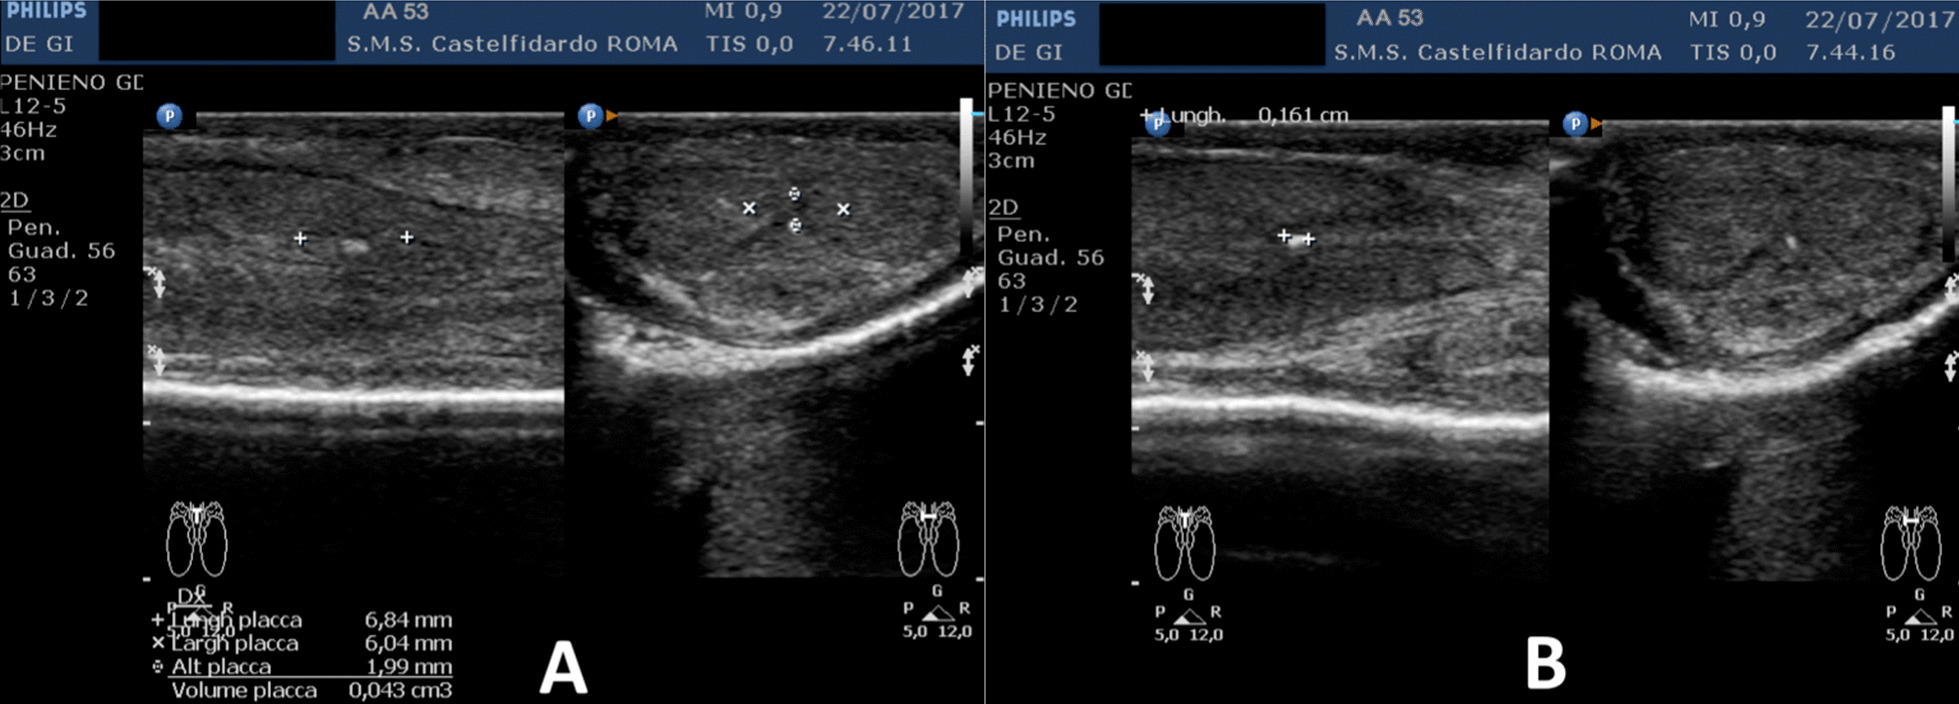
Penile ultrasound after the third treatment cycle (12 months). A Plaque measurement in longitudinal and transverse scan. B Calcification measurement in longitudinal scan
